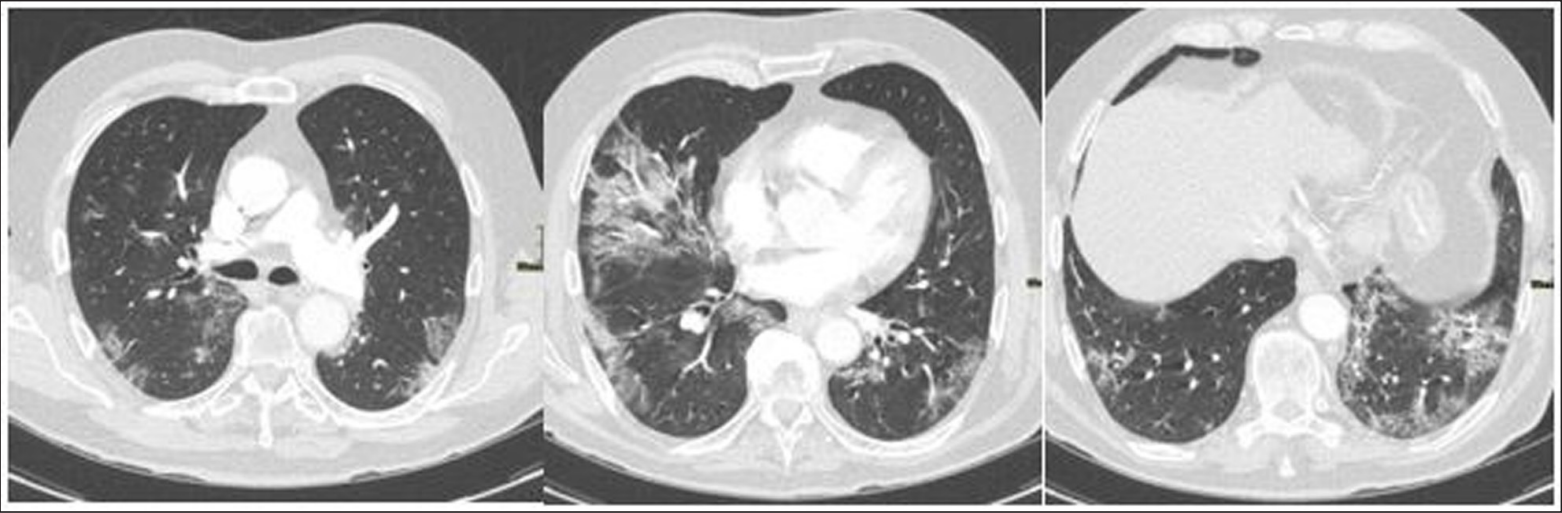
Positive CO-RADS chest CT for COVID-19 with a negative RT-PCR test: This 42-year-old patient was tested negative for COVID-19 with RT-PCR and showed multiple symptoms for COVID-19 (cough, fever, and anosmia). The chest CT showed multiple ground glass opacities and consolidation areas with crazy-paving patterns and was scored as CO-RADS 4. This patient was also considered as positive for COVID-19 by the clinician.
